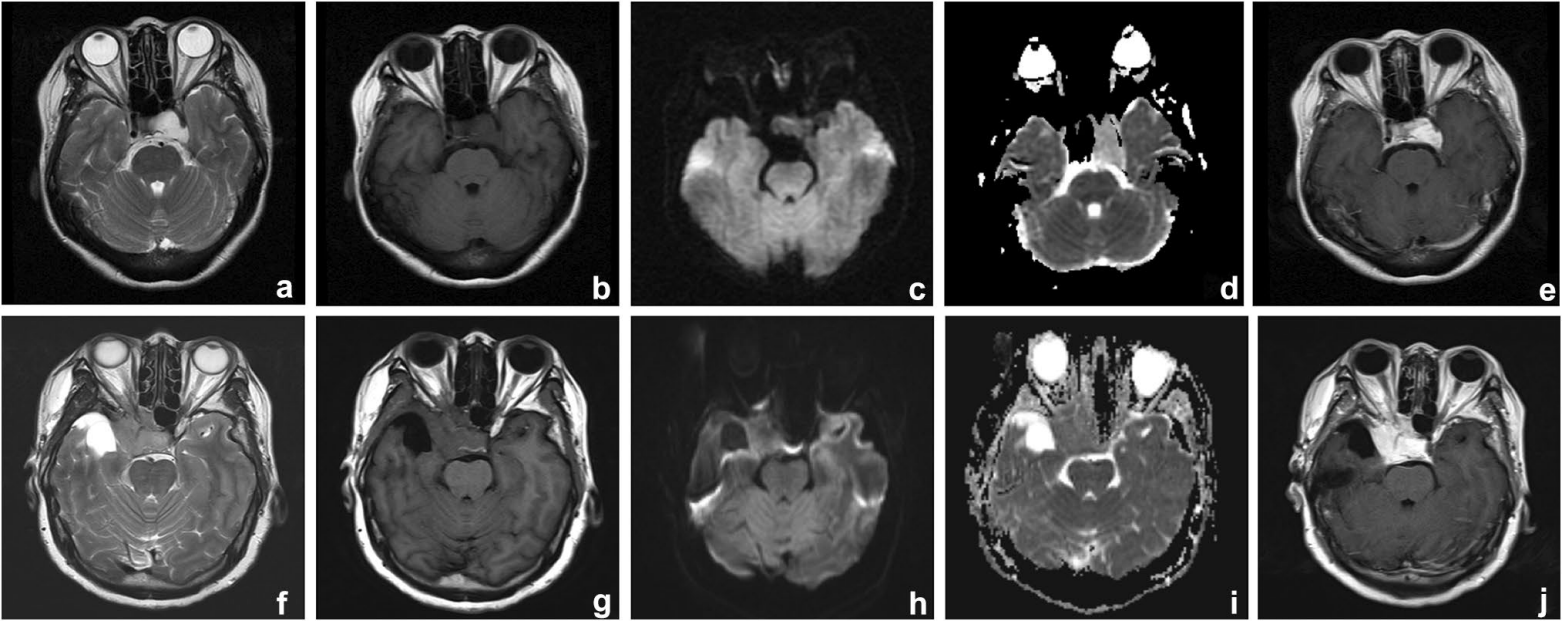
(a–e) Images of parasellar cavernous hemangioma in a 54-year-old woman. (f–j) Images of parasellar meningioma in a 58-year-old woman. MRI protocol included (a, f) axial T_2_-weighted images, (b, g), axial T_1_-weighted images, (c, h) diffusion-weighted images, (d, i) apparent diffusion coefficient maps, and (e, j) contrast-enhanced T_1_-weighted images. Cavernous hemangioma exhibited hyperintensity on T_2_-weighted images, hypointensity on T_1_-weighted images, DWI, and ADC map, and CE-T_1_WI showed homogeneous enhancement. Meningioma exhibited slightly hyperintensity on T_2_-weighted images, slightly hypointensity on T_1_-weighted images, DWI, and ADC map, and CE-T_1_WI showed homogeneous enhancement.
